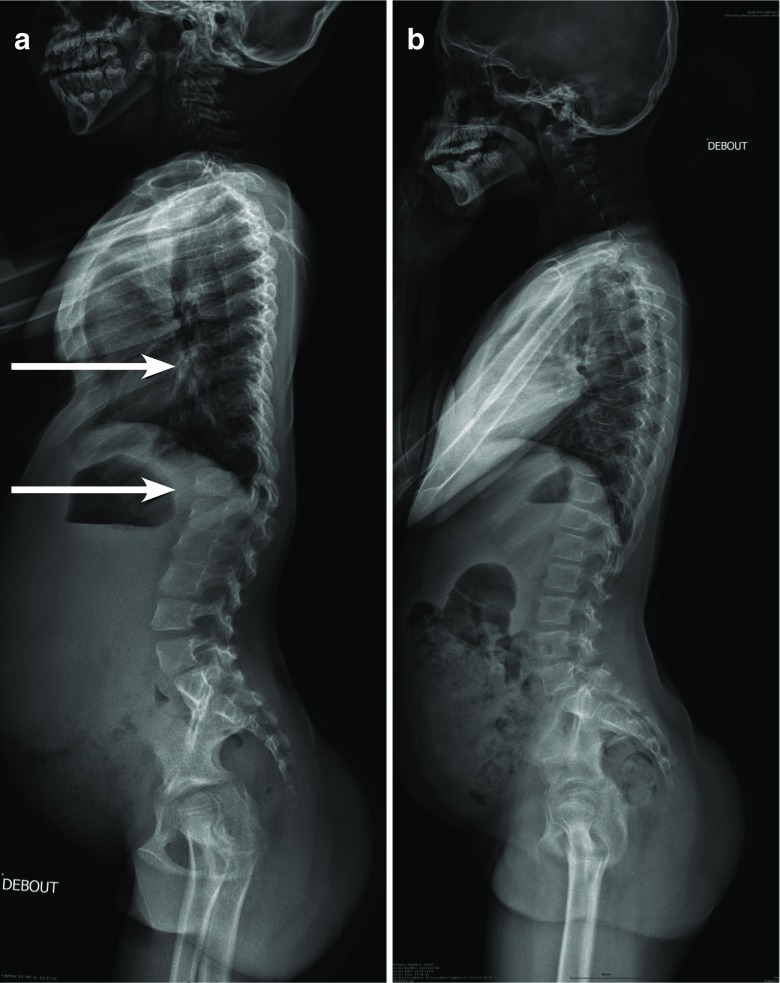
Radiographic image of the spine profile in a 7 year old child with attenuated form of MPS I (a). There is thoracic kyphosis in the thoracolumbar junction, but without the characteristic appearance of the beak-like projection generally seen on the L1 or L2. The vertebrae are discretely ovoid shape in the thoracic region. However, the same radiographic image of another child (11 years old) with attenuated MPS I does not show any anomalies (b)
